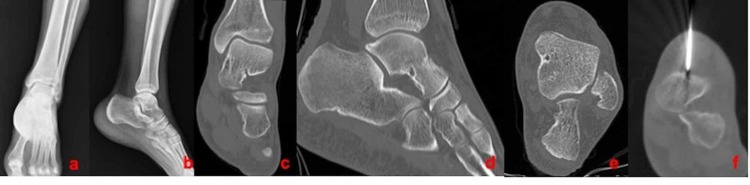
A 24-year-old male patient with OO localized in the a) talus, b) AP and lateral X-ray; c), d), and e) coronal, sagittal, and axial CT view; and f) axial CT view during the RFA procedure OO: osteoid osteoma; AP: anteroposterior; CT: computed tomography; RFA: radiofrequency ablation
